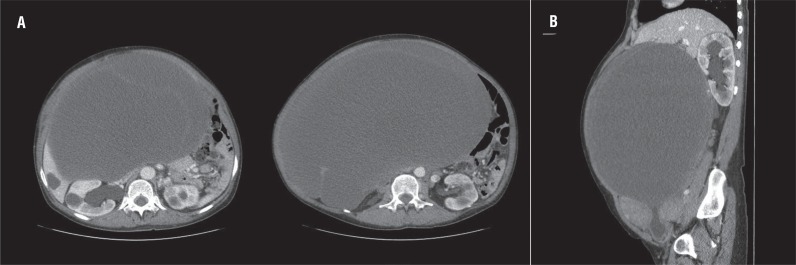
The axial (A) and sagittal scan (B) of contrast-enhanced abdominopelvic computed tomography show the hydronephrosis caused by a large cystic mass in the abdominopelvic cavity.
